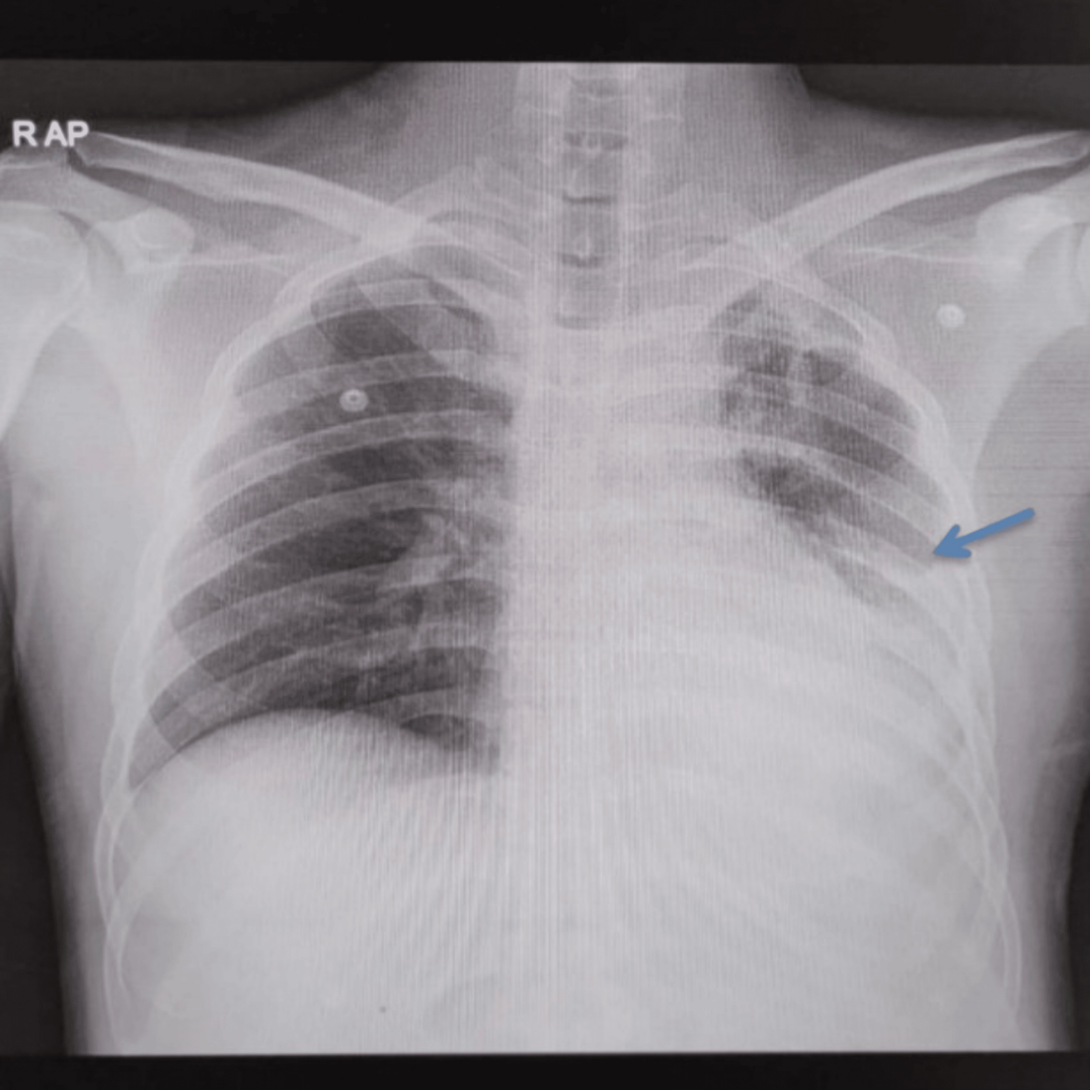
Chest X-ray (AP) revealing significant resolution of REPE and near-complete re-expansion of the left lung (arrow) AP: anteroposterior
